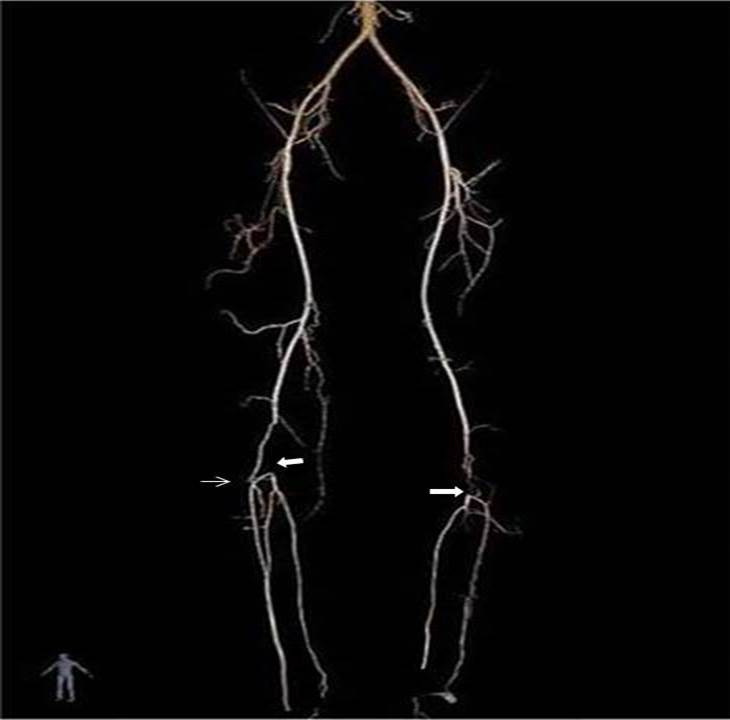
Computed tomography angiography of lower limbs: Bilateral popliteal occlusions (white arrows) and opacifications can be seen.
